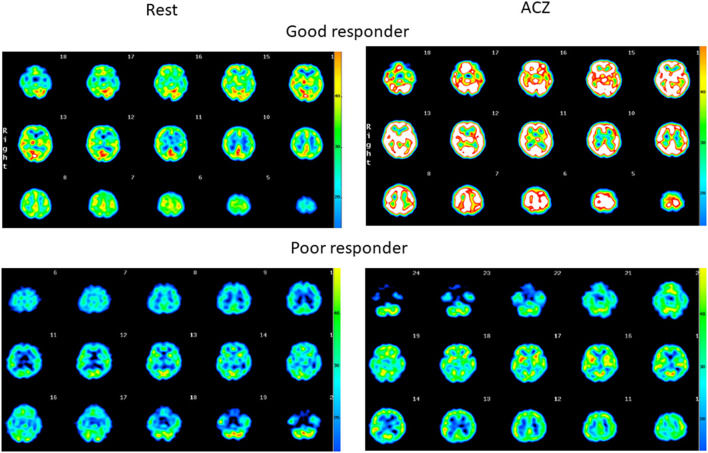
Quantitative SPECT images at rest and after ACZ injection. (Upper) Diffuse increase in the rCBF, indicating the maintenance in cerebrovasoreactivity in the patient (64-year-old female without stroke). (Lower) Only a slight increase in the rCBF, indicating little residual cerebrovasoreactivity in the patient (42-year-old male with stroke).
